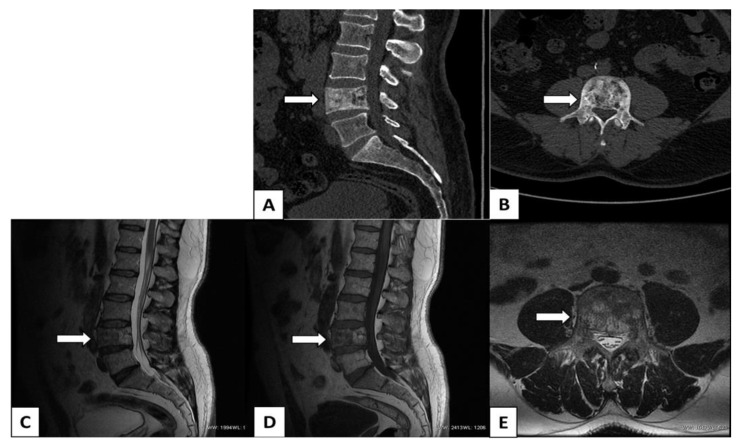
Computed tomography angiography (CTA) and magnetic resonance imaging (MRI): (A) Sagittal CT image, bone window, reveals mildly enlarged and “squared” L4 (arrow) vertebral body. The vertebral body is mostly sclerotic with cortical thickening and disorganized, coarsened vertical trabeculae. (B) Axial CT image, bone window, shows heterogenous sclerotic remodeling and end enlargement of the L4 vertebra with sclerotic cortical thickening. Changes also affect the vertebral pedicles and the neural arch of the vertebra. (C,D) Sagittal MRI T1-weighted image (T1WI) and T2WI of the lumbar spine reveal enlargement of the L4 vertebral body with mild vertebral canal stenosis. The L4 vertebral body is “squared” and shows loss of the anterior concave margin. Heterogeneous change of bone marrow signal of the affected vertebral body. In the anterior part, the low signal on T1WI and T2WI suggests sclerosis-fibrosis and late, blastic inactive phase of Paget’s disease of bone (PDB). In the posterior part, the high signal on T1WI and T2WI suggests fatty marrow of mixed (second) phase of PDB. (E) Axial MRI T2WI image shows an enlargement of the vertebral body and pedicles, heterogeneous bone marrow remodeling with thickened trabeculae, and patchy areas of high signal in the posterior part, suggesting fatty transformation.
